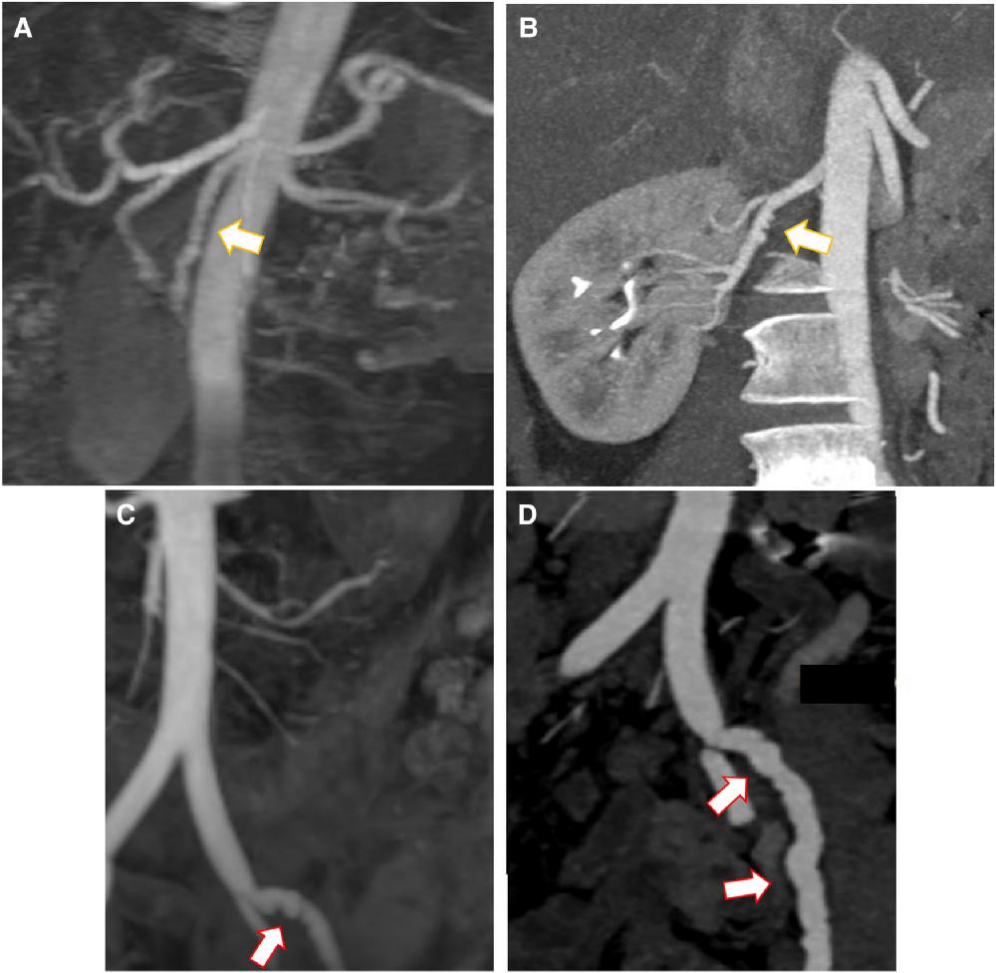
Fibromuscular dysplasia in proximal renal artery; comparison between contrast-enhanced magnetic resonance angiography (*A*) and computed tomography angiography (*B*). Fibromuscular dysplasia in iliac artery; comparison between contrast-enhanced magnetic resonance angiography (*C*) and computed tomography angiography (*D*).
